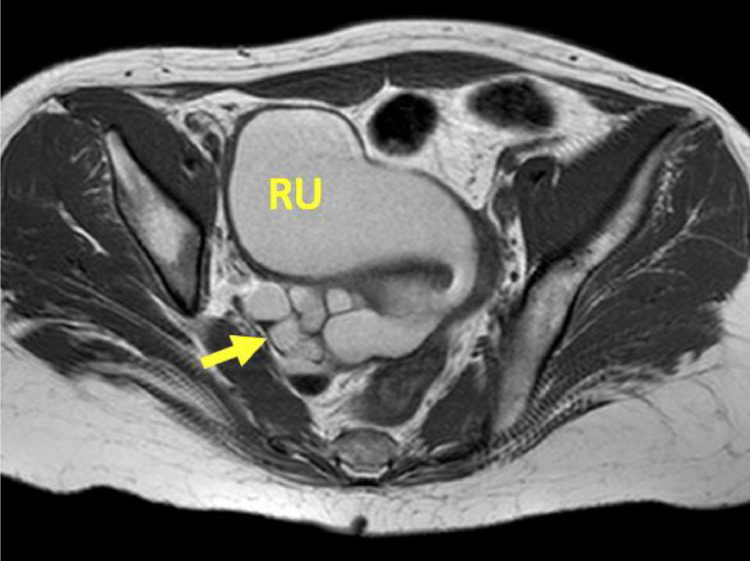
MRI T1-Weighted axial. Large serpiginous, tubular structure (arrow) in the right adnexal region with septa representing a dilated right fallopian tube. There are dependent T1 hyperintense products and hypointense fluid levels in the endometrial cavity and fallopian tube compatible with chronic blood products.
